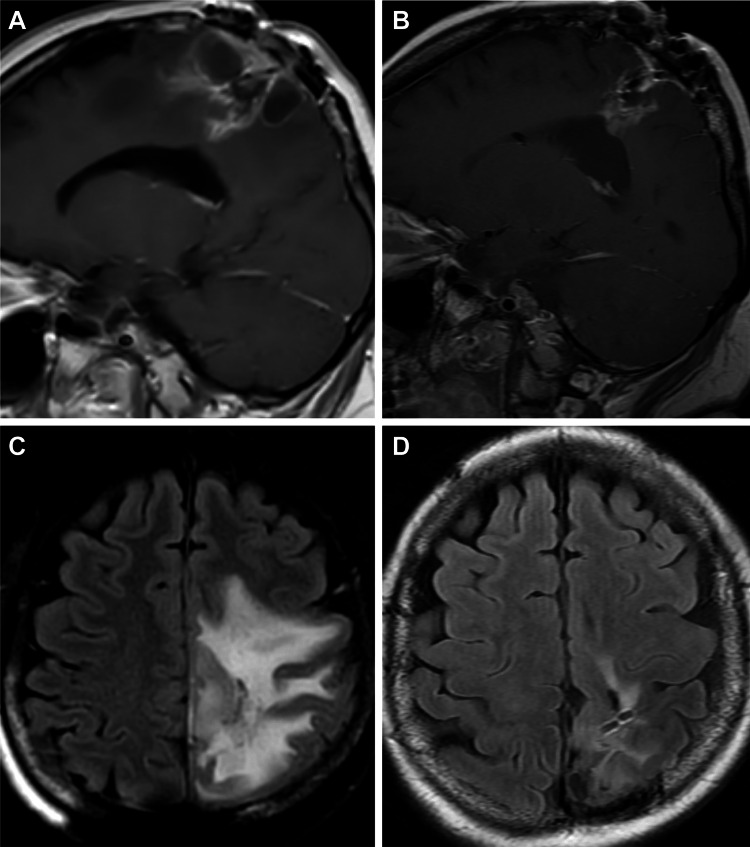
MRIs for patient 10 Patient 10 had continued disease progression after a second surgical resection as evidenced by increasingly nodular enhancement (A, sagittal magnetic resonance imaging [MRI]) and progressive cerebral edema (B, axial MRI). The patient subsequently initiated ketogenic metabolic therapy, and imaging obtained at the next follow-up evaluation (four months later) showed significant improvement in lesional enhancement (C, sagittal MRI) and markedly reduced cerebral edema (D, axial MRI). Used with permission from Barrow Neurological Institute, Phoenix, Arizona.
